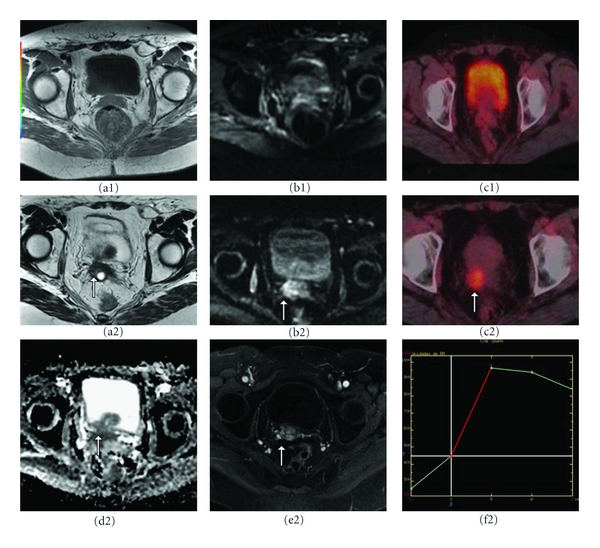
Proven vaginal fornix recurrence after radical surgery for cervical cancer in a 65-year-old woman. *First row *(a1)* axial T1-WI*, (b1)* DWI and *(c1)* PET-CT images* show no remarkable alteration except for an abnormal signal at DWI in the right vaginal fornix (white arrow), with an abnormal ADC value, measuring 1.3 × 10^−3^ mm^2^/s. *Second and Third rows*: six months later (a2)* axial T2-WI*, (b2)* DWI*, (c2)* PET-CT*,* and *(d2)* ADC map show that* the ADC value in the right vaginal fornix had decreased to 1.1 × 10^−3^ mm^2^/s, corresponding to a hypermetabolic focus at PET-CT (white arrow). (e2)* and *(f2)* DCE-MRI* depicted a small focus with faster first-pass contrast agent enhancement, with a type III kinetic curve (washout type), which is indicative of malignancy.
